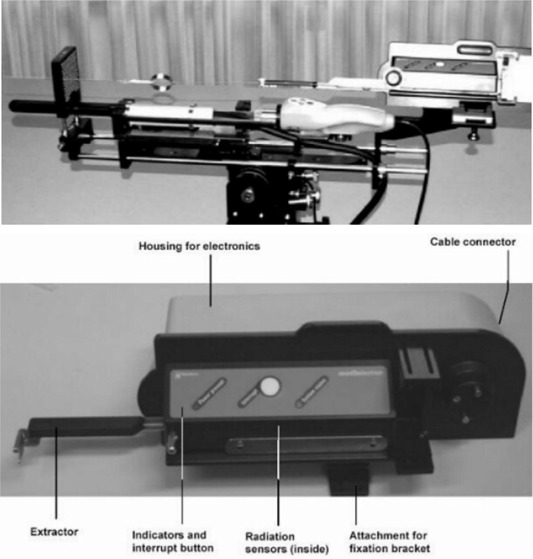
(a) The complete assembly of the image acquisition system and seedSelectron. Left to right: template, bimodal ultrasound probe, ultrasound probe stepper assembly, endocavity rotational mover (ECRM), and the seedSelectron. (b) closeup of the Nucletron seedSelectron.
